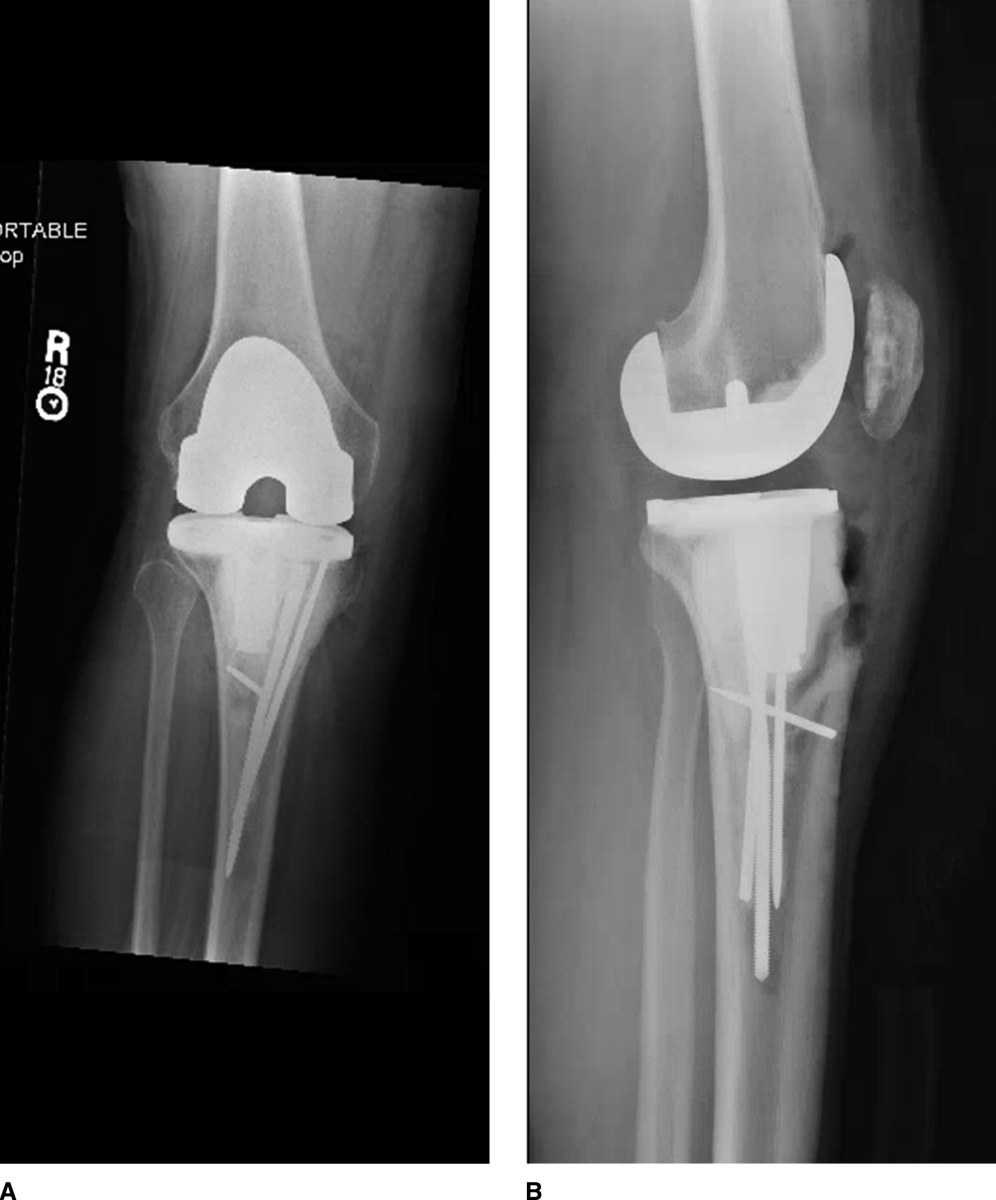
AP (A) and lateral (B) postoperative radiographs following revision of the tibial component with curettage, argon beam coagulation, cementation, Steinmann pin fixation, and extensor mechanism reconstruction.
